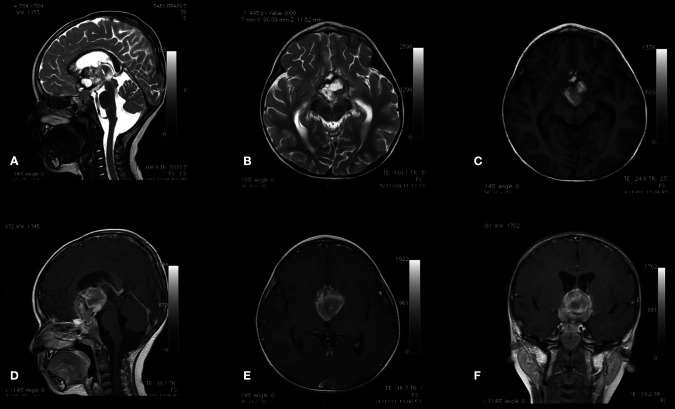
Sagittal (A) and axial (B) T2-WI and axial (C) T1-WI MRI scan sequences of the brain reveal large sellar and suprasellar cystic and solid lesions containing subacute components of blood products. The lesion is enhanced heterogeneously following intravenous contrast administration (D–F).
